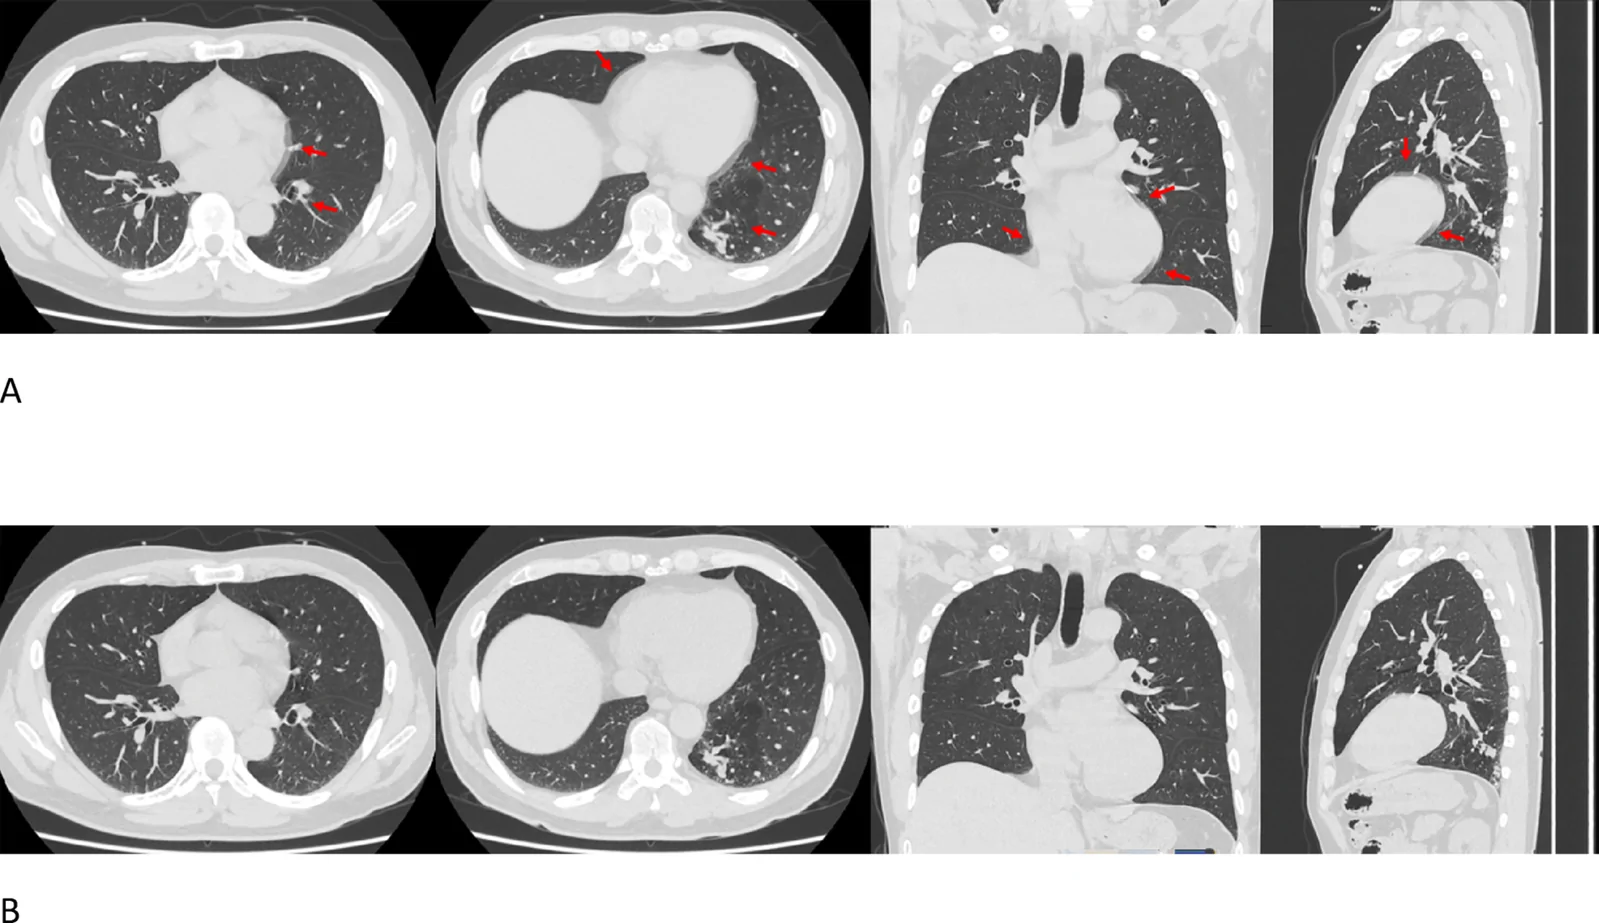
A 42-year-old male with pulmonary sequestration. A: (L to R: axial CT (cranial to caudal), coronal multiplanar reformatted (MPR), and sagittal MPR images from CT data without CLEAR Motion). Axial CT images and coronal and sagittal MPR images show cardiac motion artifacts (red arrows) at the cardio-pulmonary borders in both lungs. B: (L to R: axial CT (cranial to caudal), coronal multiplanar reformatted (MPR), and sagittal MPR images from CT data without CLEAR Motion). All cardiac motion artifacts clearly disappeared, and the borders between the sequestrated lung and normal lung, pulmonary vasculatures, and bronchi were clearly depicted
